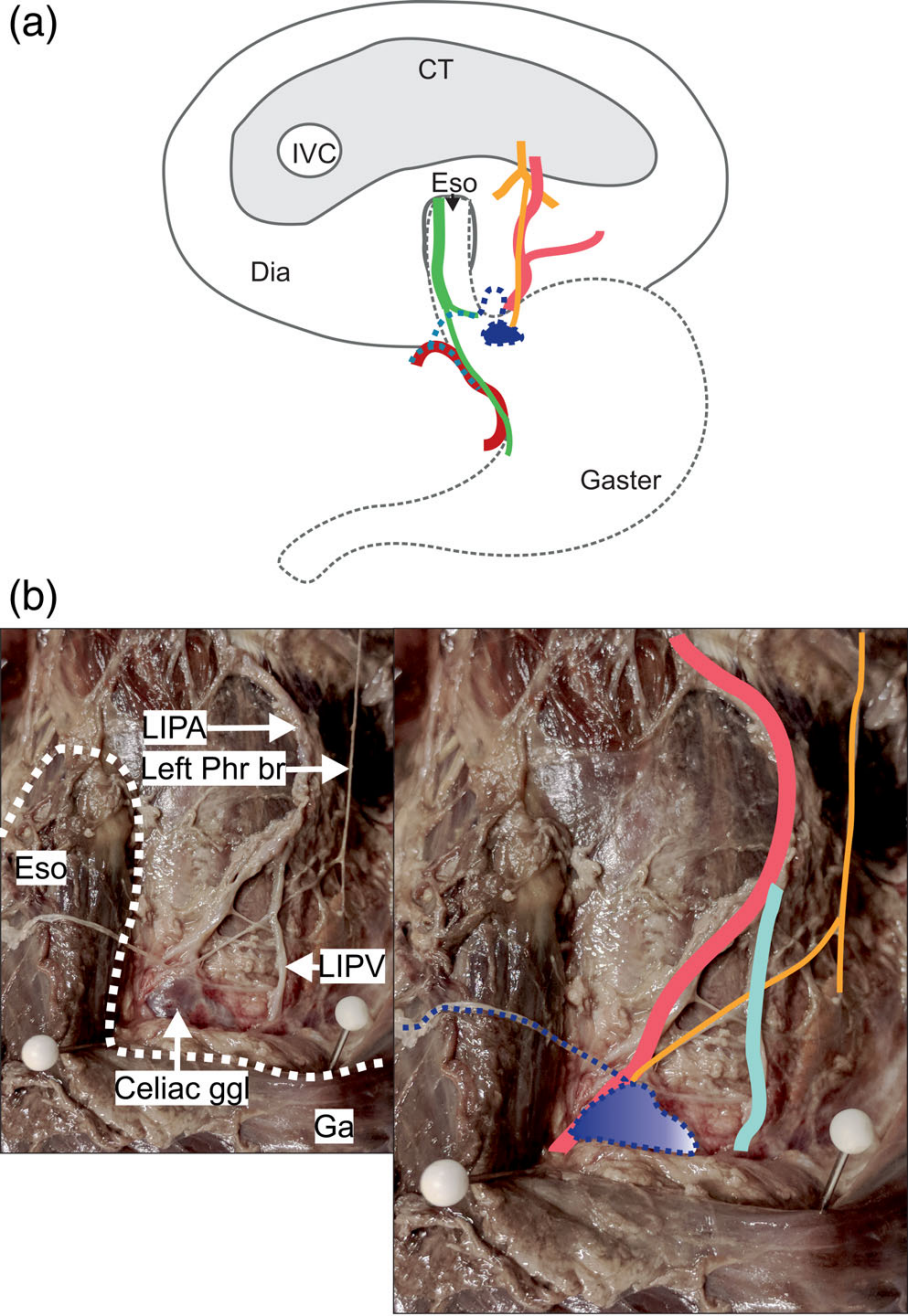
A distinct branch of the left phrenic nerve directly connects to the celiac ganglion (Variant C, *n* = 3). (a) The phrenic nerve branch (yellow) connects to the left‐sided celiac ganglion (dark blue). A celiac plexus branch (dark blue dotted) forms a wide loop in front of the esophagogastric junction and connects to other plexuses. No arterial branches of the left inferior artery (light red) accompany the nerve. (b) The white dotted line surrounds the esophagus and the proximal part of the gaster (left picture). The left phrenic branch (yellow) connects to the left‐sided celiac ganglion (dark blue). The anatomical structures are highlighted in color (right picture). CT = central tendon; Dia = diaphragm; Eso = esophagus, Ga = gaster; ggl = ganglion; IVC = inferior caval vein; LIPA = left inferior phrenic artery; LIPV = left inferior phrenic vein; Phr br = phrenic nerve branch. Color code: green: anterior vagal trunk with gastric branches, yellow: phrenic nerve with muscular branches and branch to the ganglion; dark blue dotted: left celiac‐nerve branches; light blue dotted: left gastric plexus; dark red: left gastric artery; light red: left inferior phrenic artery; light blue: left inferior phrenic vein. [Color figure can be viewed at http://wileyonlinelibrary.com]
